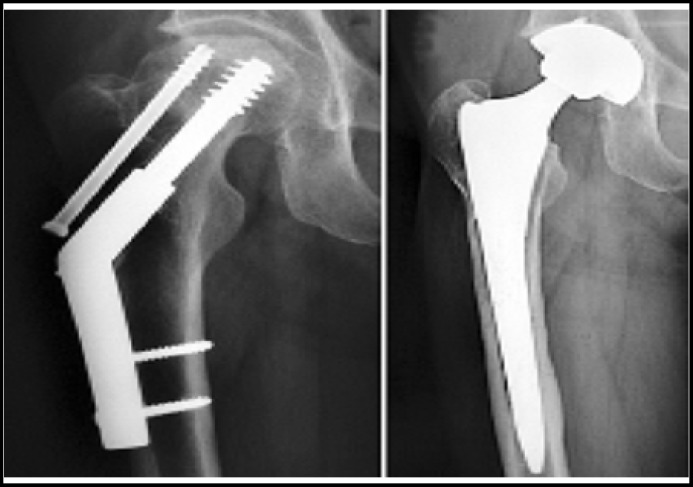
Postoperative X-ray of endoprosthetic replacement of patients with failed treatment of intertrochanteric hip fractures
